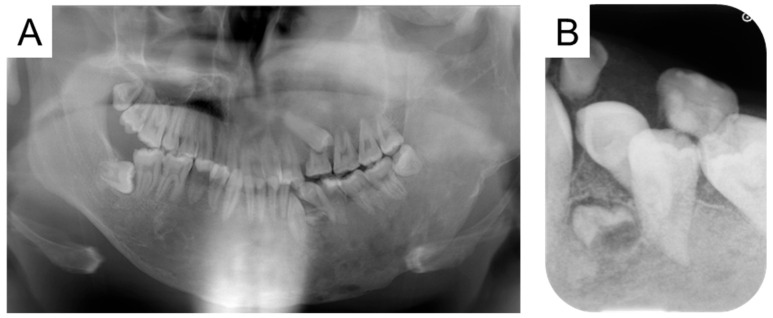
Radiographic examination at the age of 23 years and 11 months ((A): Panoramic photograph, (B): Periapical photograph).
